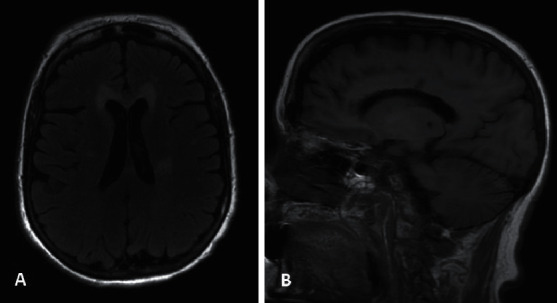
(a) An axial fluid-attenuated inversion recovery (FLAIR) sequence demonstrating a hyperintense lesion in the genu of the corpus callosum and smaller lesions, most notably in the left corona radiata area. (b) A sagittal T1-weighted image demonstrating a hypointense lesion in the genu and body of the corpus callosum.
